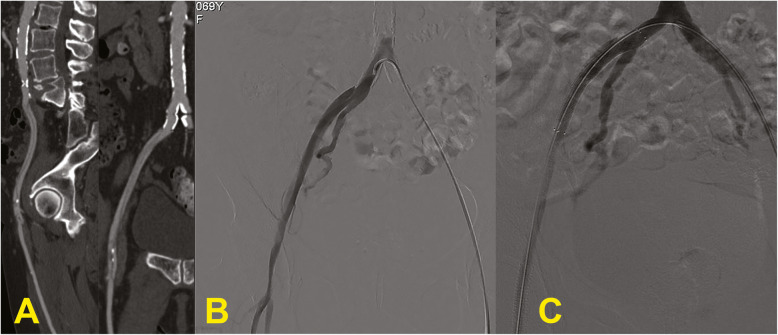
a-c Dissection. Sagittal and coronal CT reformats (a) show dissection of the whole iliac axis extending up to the common femoral artery. Angiography with crossover approach (b) confirms the dissection with iliac stenosis proximally to the femoral bifurcation. The dissection has been treated with the placement of two stents; angiography after treatment (c) demonstrates restoration of regular patency of iliac and common femoral arteries
